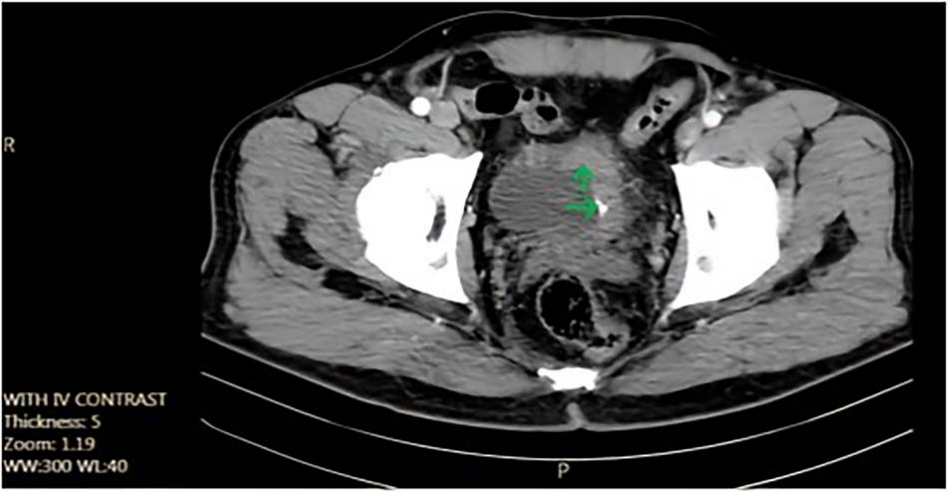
Axial pelvic CT scan shows large urinary bladder tumor (arrow).
